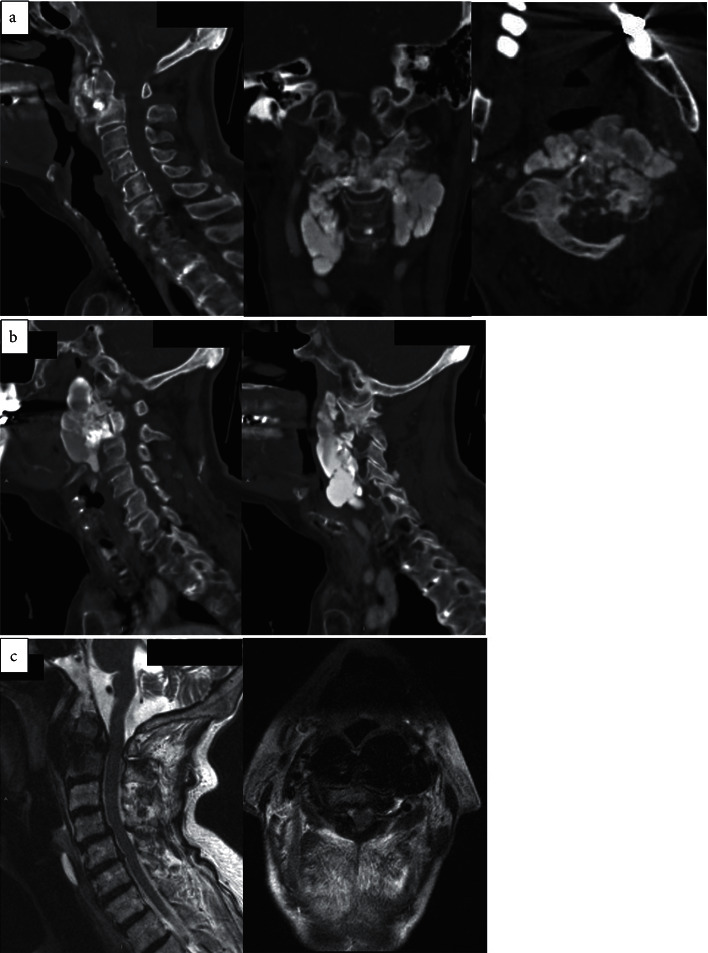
Noncontrast CT scans and T2-weighted MRI of preoperative odontoid fracture with associated tumoral calcinosis. (a) Sagittal, coronal, and axial CT images, respectively from left to right, show pathological type II odontoid fracture, (b) left: left parasagittal CT image shows extensive tumoral calcinosis; right: right parasagittal CT image shows extensive tumoral calcinosis, and (c) left: T2-weighted sagittal MRI shows spinal cord compression at C1-C2; right: T2-weighted axial MRI at C1-C2.
